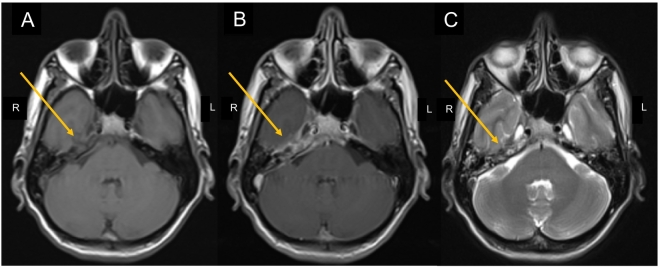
(A) MRI T1-Weighted image showing hypointense right petrous apex as compared to the left. (B) On enhanced axial T1-Weighted MRI image, prominent enhancement is seen on right petrous apex. There is also mild dural contrast enhancement in the temporal region without evidence of leptomeningeal involvement or parenchymal involvement. (C) MRI T2-Weighted image showing hyperintense right petrous apex in comparison with the contralateral petrous apex.
